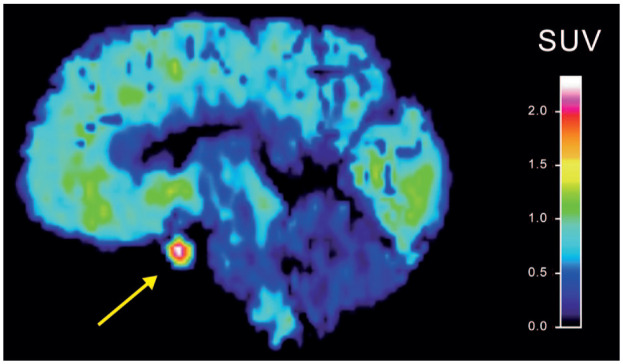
SUV image of a representative baseline PET examination showing high [^11^C]AZ10419369 uptake in the pituitary region.
